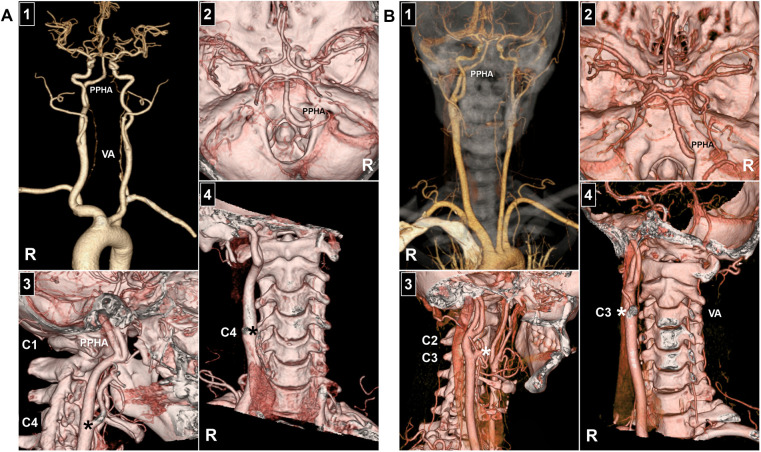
Development of VA and PcomA in PPHA. (A) Panel 1: Head and neck CTA reveals a right PPHA with bilateral VA hypoplasia and absence of PcomAs. Panel 2: Head CTA demonstrates a right PPHA coursing through the hypoglossal canal to replace the ipsilateral VA and join the basilar artery. Panels 3–4: Neck CTA images show the PPHA originating from the C1 level of the ICA, with the carotid bifurcation located at the C4 level (asterisks). (B) Panel 1: Head and neck CTA reveals a right PPHA with bilateral VA hypoplasia and absence of PcomAs. Panel 2: Head CTA demonstrates a right PPHA passing through the hypoglossal canal to replace the ipsilateral VA and join the basilar artery. Panels 3–4: Neck CTA images show the PPHA arising from the C2 level of the ICA, with the carotid bifurcation at the C3 level (asterisks). C1, C2, C3, C4: first, second, third, and fourth cervical vertebrae, respectively; CTA, computed tomography angiography; ICA, internal carotid artery; PcomA, posterior communicating artery; PPHA, persistent primitive hypoglossal artery; R, right; VA, vertebral artery.
